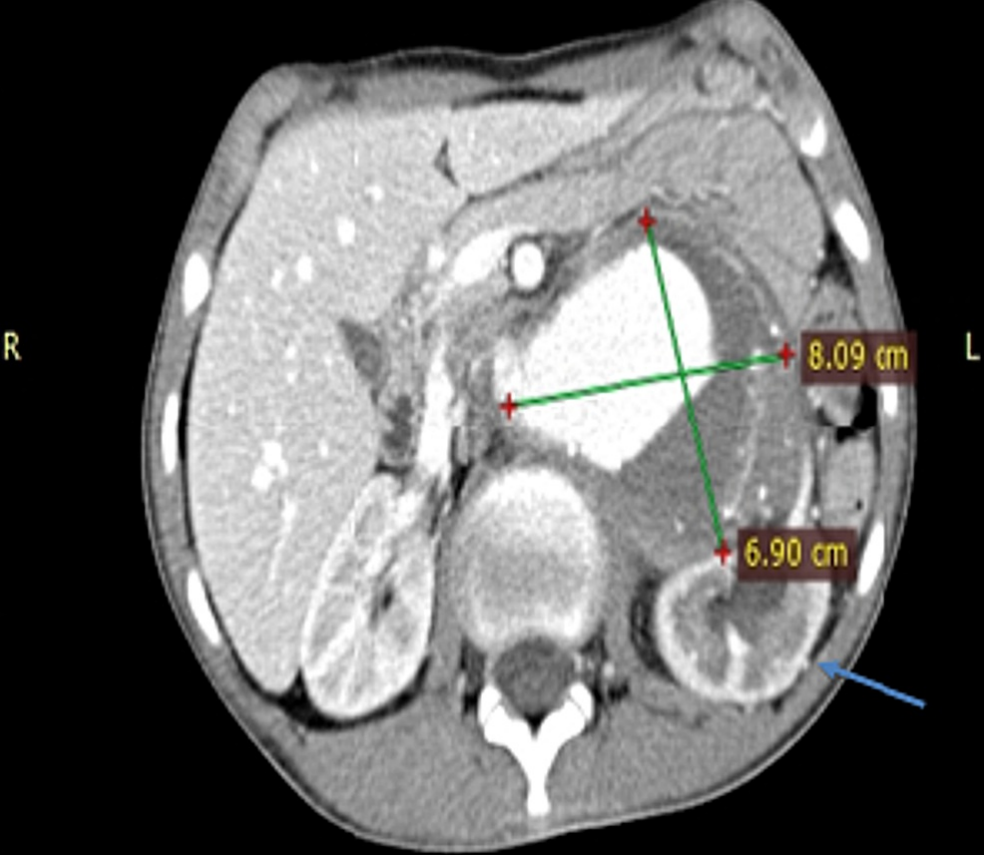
CT angiogram axial view showing the pararenal component of the aneurysm to the largest size. The left renal artery is not visible and the left kidney is also pushed down by the aneurysm (shown by blue arrows)
